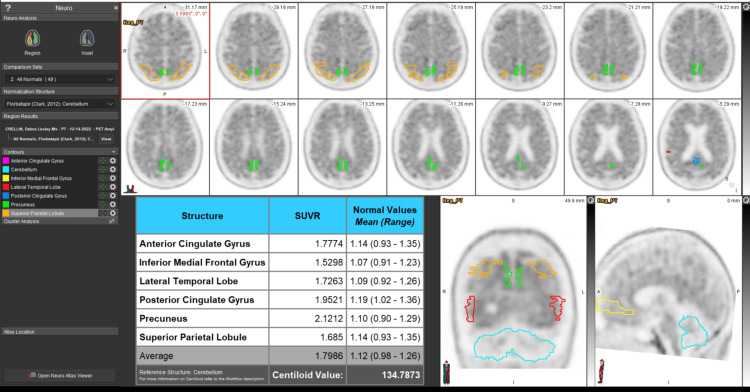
Pathological SUVR of tracer in the cingulate, medial frontal, lateral temporal, precuneus, and superior parietal lobes, which demonstrates the pattern of deposition of Aβ amyloid. SUVR: standardized uptake value ratio. The image was published with the permission of the original publisher and with a Creative Commons License. Image credits: Case courtesy of Dr. Sally Ayesa, Radiopaedia.org, rID: 166985.
